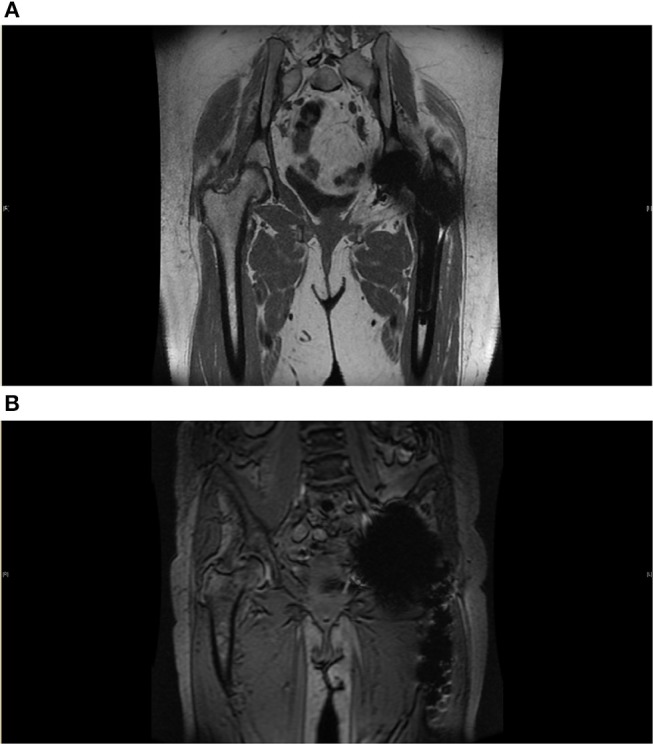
(A) Metal Artifact Reduction MRI image. Reduction sequence is utilized here in assessment of prosthesis failure in the left side following THR. Some metal artifact remains. There is no significant joint effusion, soft tissue oedema or collection seen. Common findings following THR can be seen here, this includes fatty atrophy in the short external rotators and adductor brevis. (B) Metal artifact reduction MRI image. Here, reduction sequence is utilized in order to minimize aberrations from acting as noise in the assessment of the contralateral joint. There is moderate degenerative change in the superior aspect of the right hip with near complete loss of articular cartilage and bone marrow oedema consistent with osteoarthritis. There is no hip effusion or synovitis.
